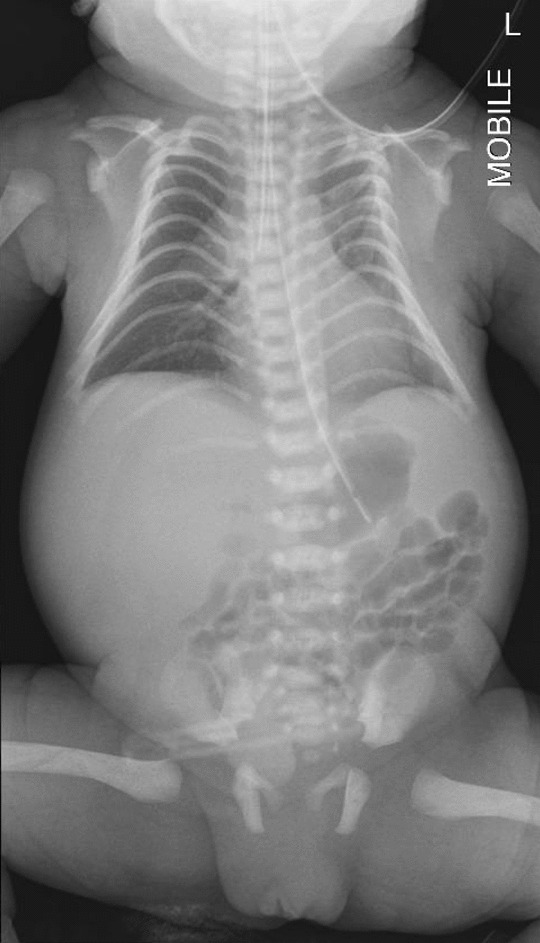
Chest X-ray showing “coat hanger” ribs
